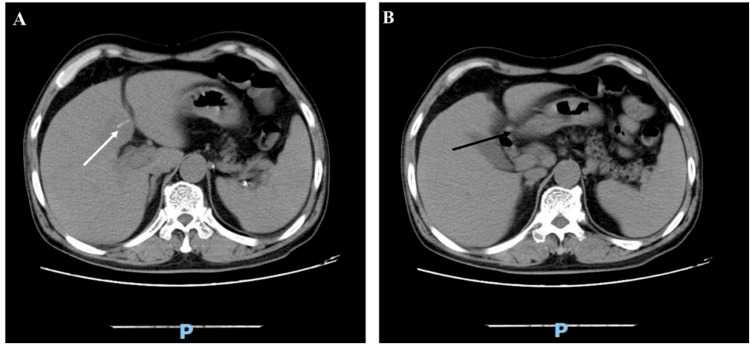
Abdominal computed tomography scan. A: A hyperdense linear body (white arrow) was found in the liver abscess. B: This linear body was attached to the stomach antrum (black arrow).
